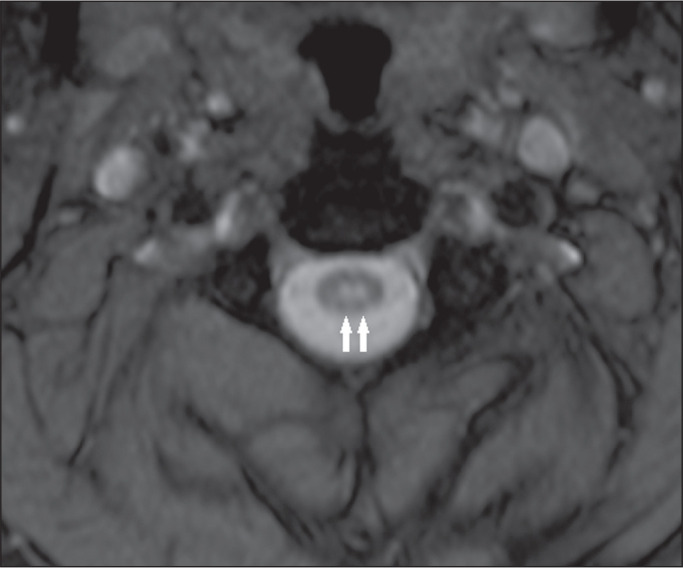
Poliomyelitis-like syndrome in a 5-year-old male. Axial T2-weighted MRI
